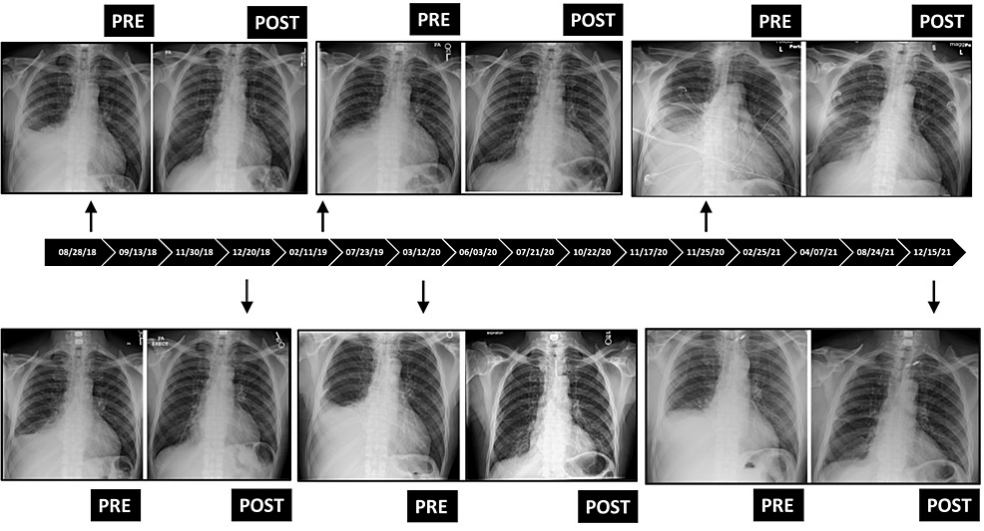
Sample chest X-rays of the patient pre- and post-thoracentesis over a four-year span highlighting the resolution of the right-sided pleural effusion. Timeline reveals the dates when each thoracentesis was performed.
